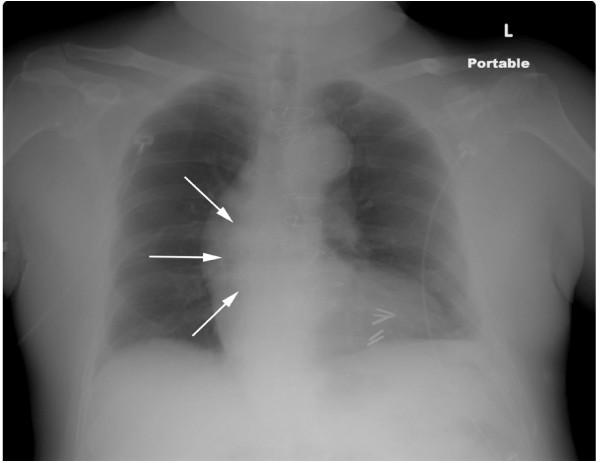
Portable chest radiograph demonstrating a 4.4 cm mass at the right AV groove as a double density (white arrows) of the ascending aorta.
